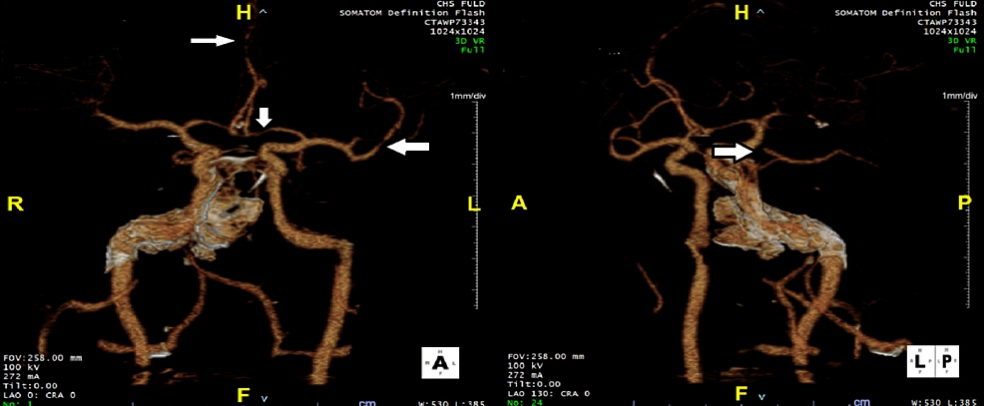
Initial computed tomography angiography (CTA) of the brain CTA brain showing multifocal stenosis affection left M2, left A1, bilateral A3, and right P1 (arrows).
